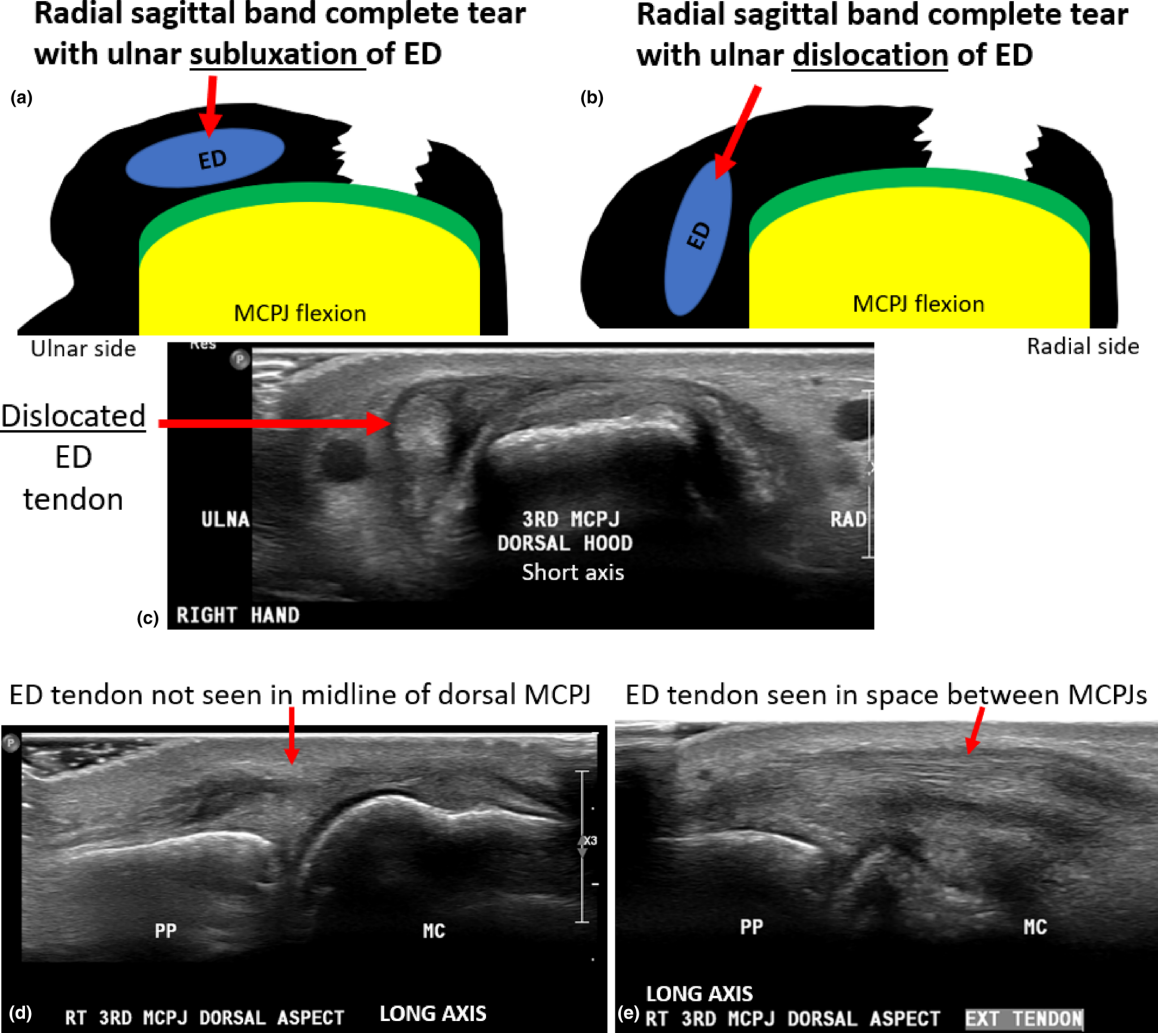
Complete tear of the radial aspect of the sagittal band. (a) Complete tear of the radial sagittal band with ulnar subluxation of the ED tendon. The ED maintains contact with the dorsal aspect of the MC head (but deviates from the ulnar dorsal aspect of midline) during MCPJ flexion. (b) Complete tear of the radial sagittal band with ulnar dislocation of the ED tendon with MCPJ flexion. When dislocated, the tendon moves into the space between MC heads and is no longer in contact with the dorsal aspect of its corresponding MC head. (c,d) Short‐axis sonographic image of the dorsal aspect of the third MCPJ. The EDC tendon is dislocated and is displaced to the ulnar aspect of the metacarpal head due to the complete tear of the radial sagittal band. (d,e) Long‐axis images over the midline dorsal aspect of the MCPJ (d) where the ED tendon is not visualised in the midline. (e) Long‐axis image acquired in the space between dorsal MCPJs where the subluxated ED tendon (subluxated in the MCPJ extension) can be seen in long axis. ED, extensor digitorum; MCPJ, metacarpophalyngeal joint; PP, proximal phalanx; MC, metacarpal; RAD, radial aspect of short‐axis image; ULNA, ulnar aspect of short‐axis image.
